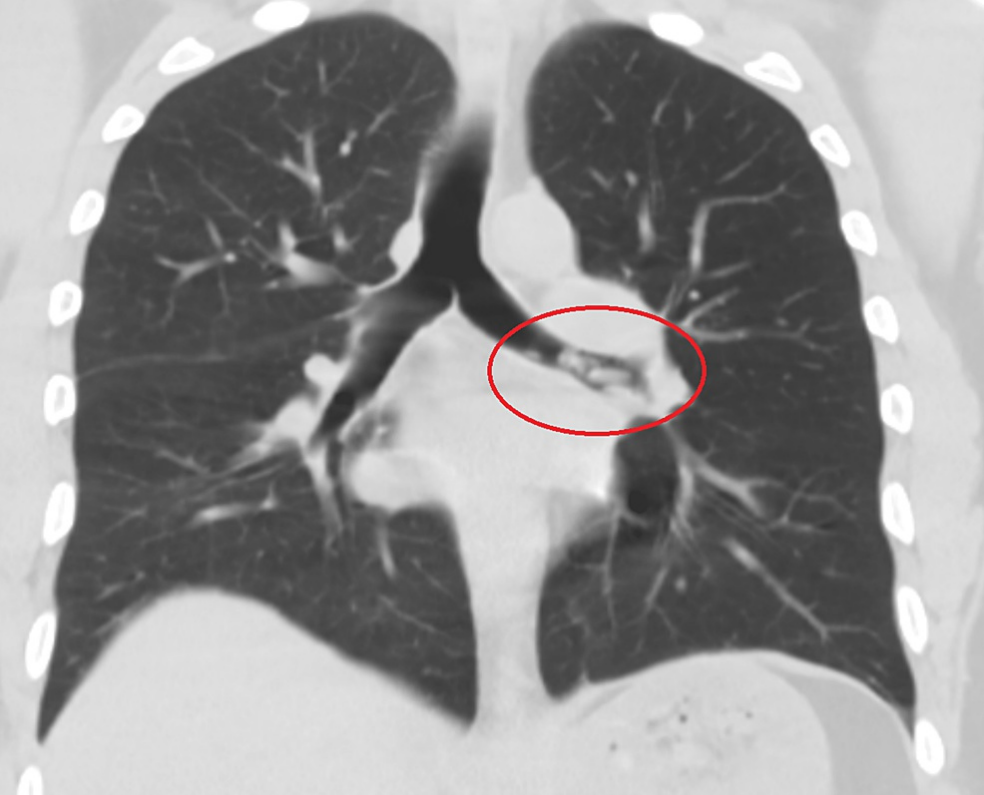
The chest computed tomography without contrast revealed multiple nodular opacities in the left mainstem bronchus with clear lungs.
